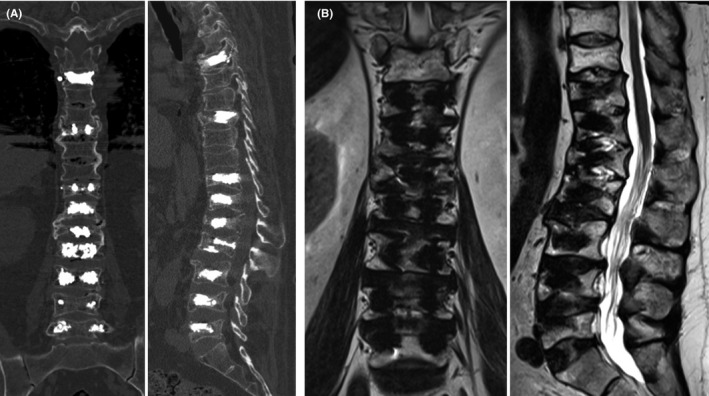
Imaging studies after the Spine‐Jack® procedure. (A, B) CT and T2‐weighted MRI show post‐procedure changes in the bodies of T5, T8, T11, T12, L1, L2, L3, L4, and L5, which correspond to the Spine‐Jack® system
